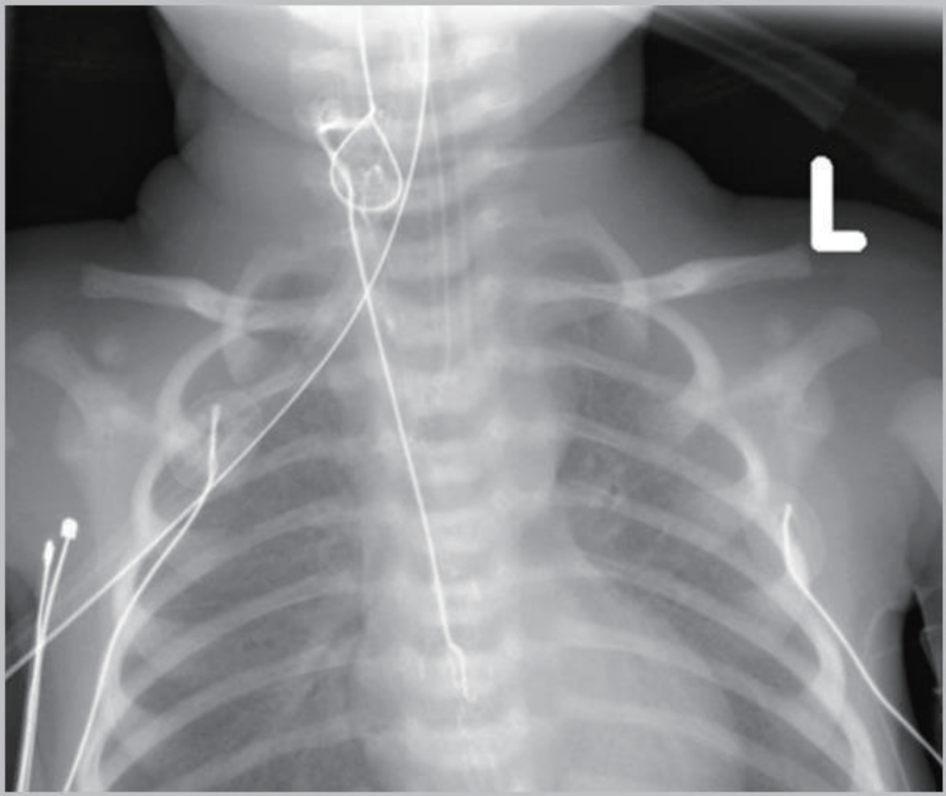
Postoperative chest radiograph obtained after attempted withdrawal of the nasogastric (NG) tube showing the NG with a knot in the proximal esophagus.
